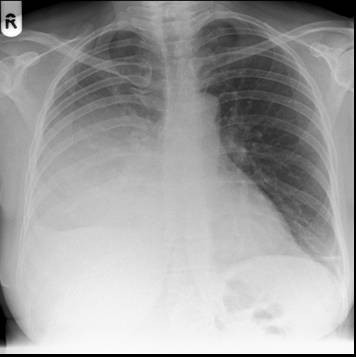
Chest X-ray on admission. This X-ray image shows a large right-sided pleural effusion.
